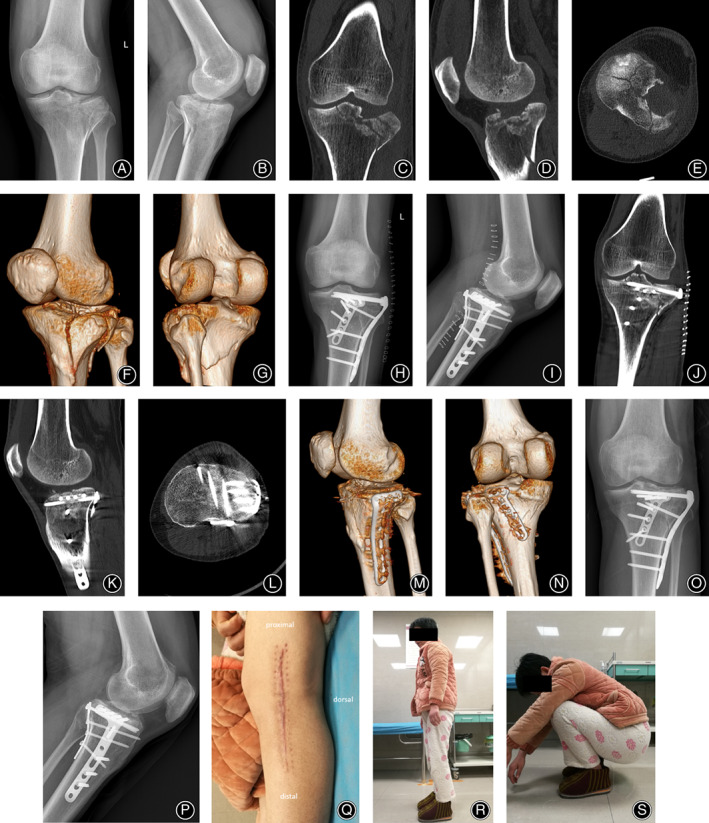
Typical case 2. A 46‐year‐old woman was injured by falling from a height. (A–G) Preoperative X‐ray and CT images show a posterolateral tibial plateau fracture combined with lateral tibial plateau fracture. The lateral tibial plateau has a split fracture with articular surface collapse, and the posterolateral tibial plateau has a posterolateral wall fracture with the fracture line extending to the posteromedial wall. (H–N) Postoperative X‐ray and CT images show an anatomical reduction of the tibial plateau fractures. (O, P) Eleven weeks after operation, the anteroposterior and lateral radiographs of the knee show that the fracture is completely healed. (Q) The appearance of the patient's surgical incision. (R, S) The function of the patient's knee joint at the last follow‐up. (In this case, the posterolateral tibial plateau fractures were mainly posterolateral wall fractures).
